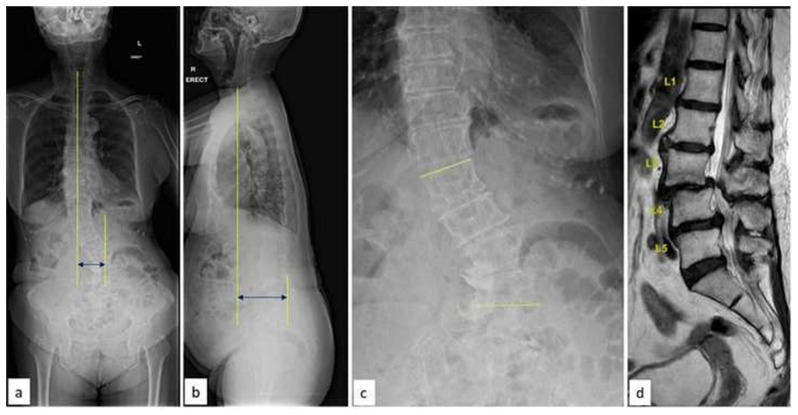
(a–b) Whole Spine Antero-Posterior and lateral radiographs showing coronal and sagittal imbalance in a patient with Degenerative lumbar scoliosis (c) AP radiograph of the lumbar spine showing the magnitude of the deformity (d) Mid-sagittal MRI showing L3–L4 anterolisthesis with multiple level disc degeneration.
